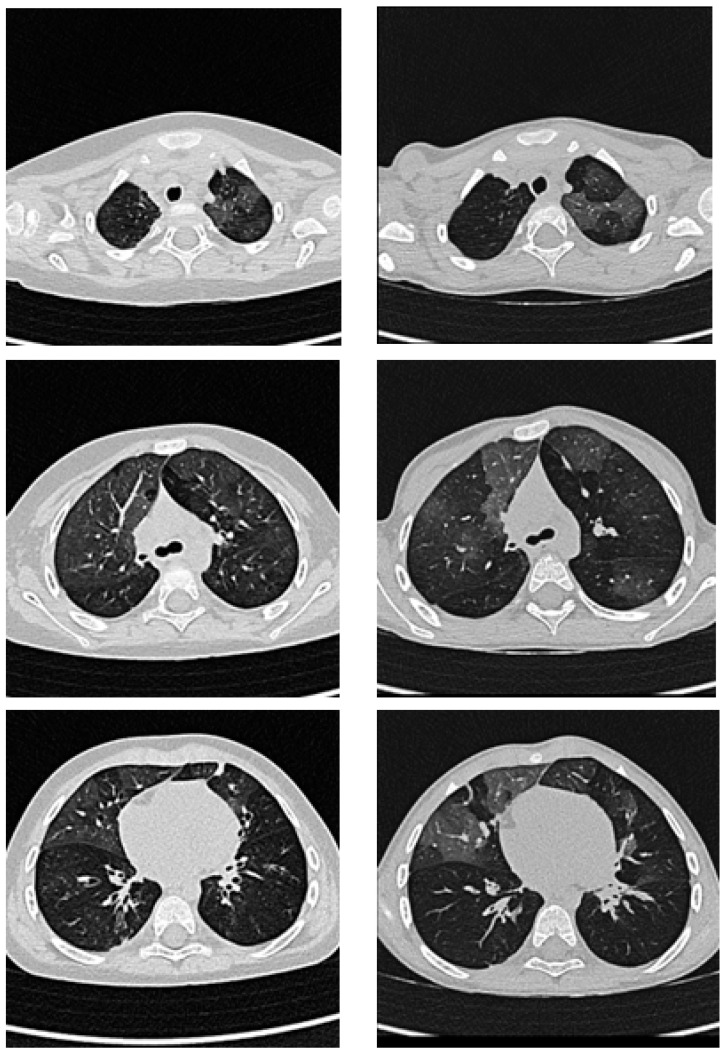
Improvement of lung involvement on contrast-enhanced chest computed tomography before and after treatment. (Left) panels: coronal chest CT images at the time of diagnosis. (Right) panels: corresponding images obtained at the same anatomical levels 24 months after treatment initiation, showing partial radiological improvement.
